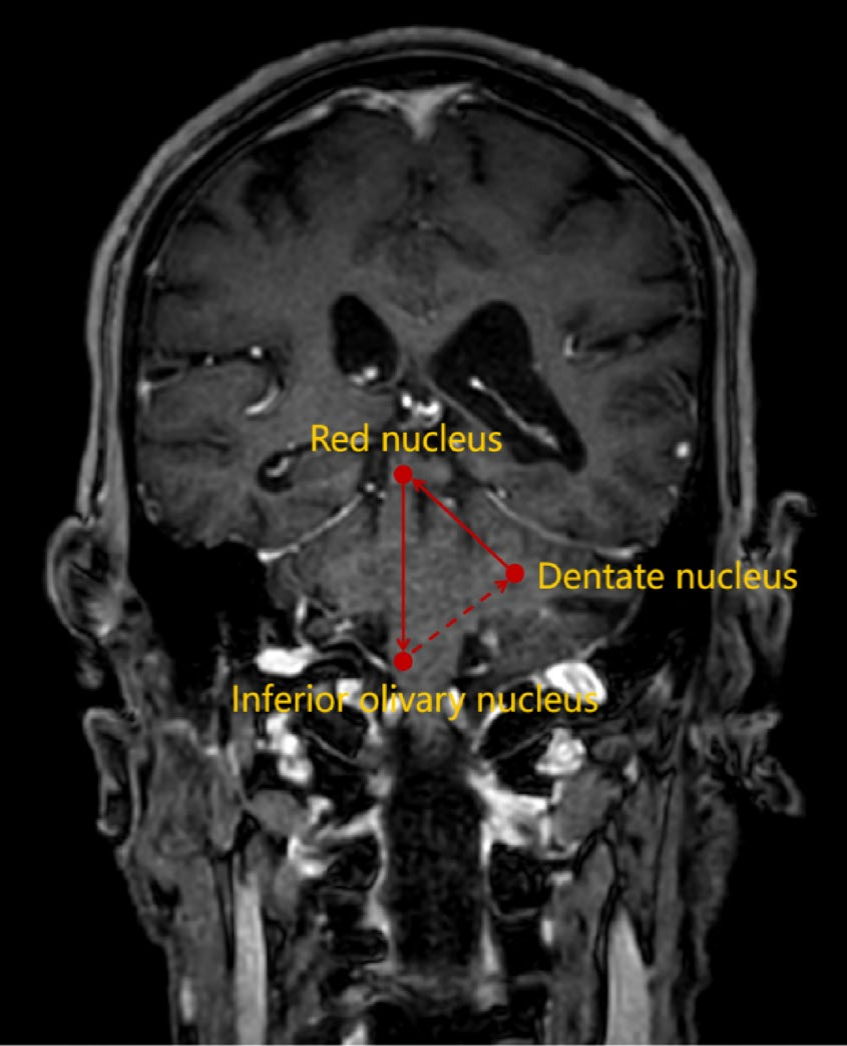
The anatomical location of the GMT on coronal cranial MRI.The GMT consists of the contralateral dentate nucleus in the cerebellum, the ipsilateral ION in the medulla oblongata, and the ipsilateral red nucleus at the level of the midbrain.
